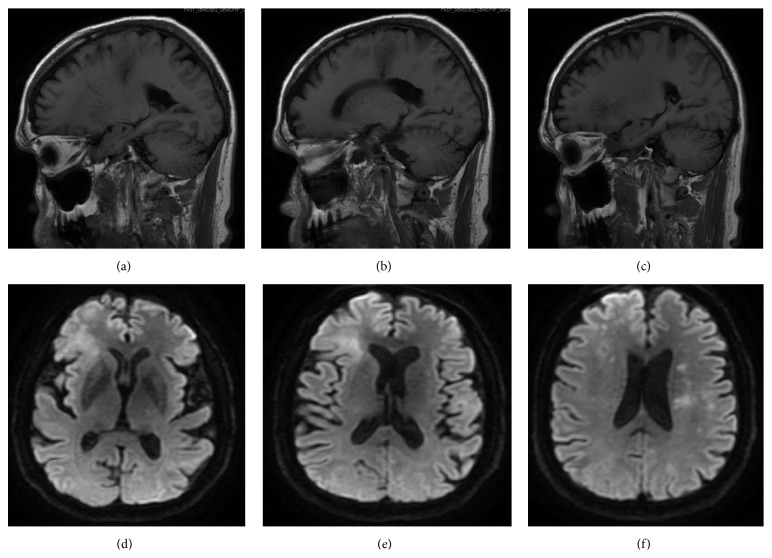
T1-weighted sagittal images (a–c) and diffusion-weighted images (DWI) (d–f). Images progress from right to left from (a) through (c). Images progress superiorly to inferiorly from (d) through (f).
